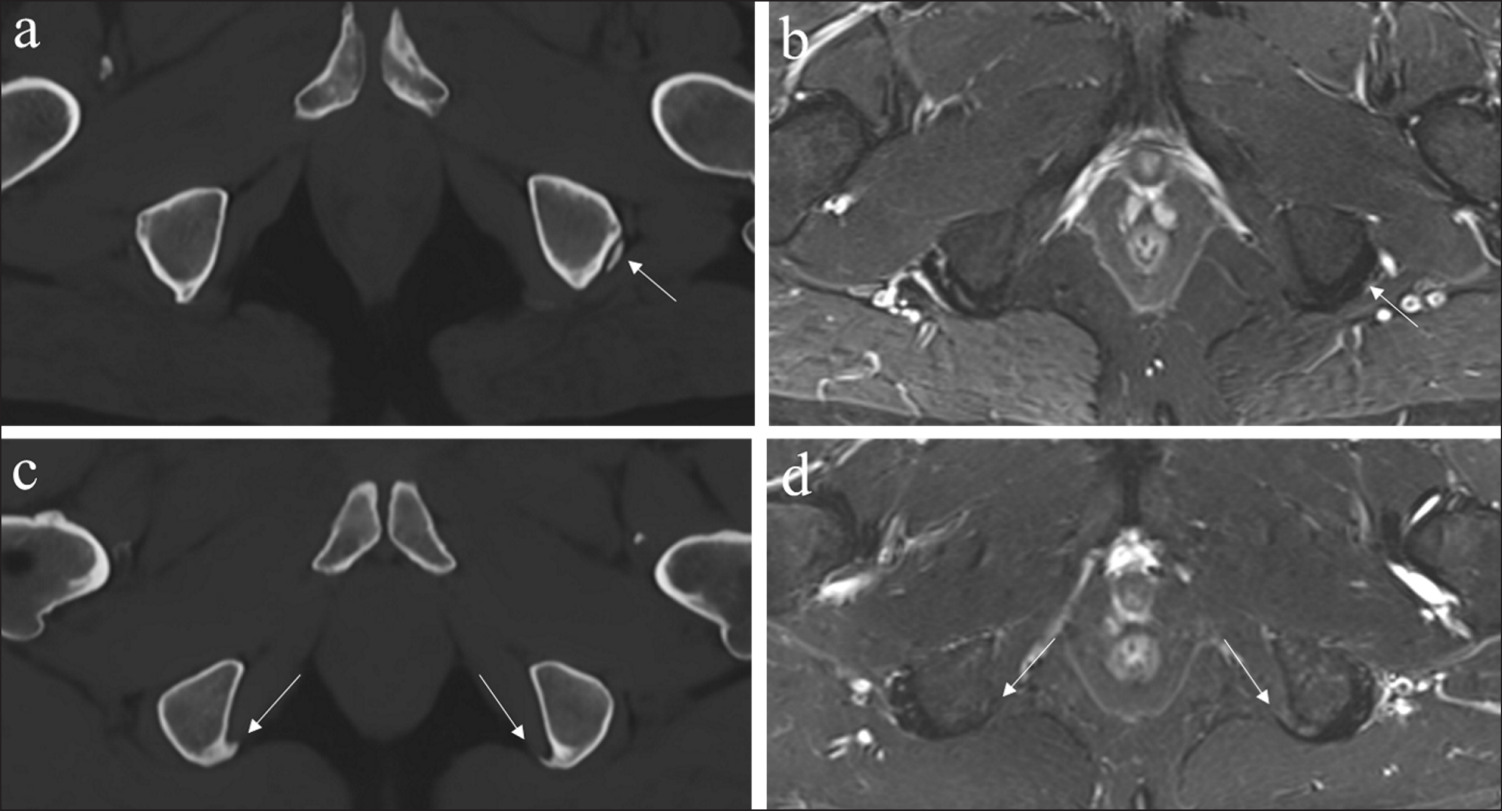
Incidental findings on sacroiliac joint MRI, depicted on synthetic CT images, but hard to appreciate on the T2 Short TI Inversion Recovery (STIR) images. (a, b) Old avulsion of the left ischial tuberosity in a 47-year-old male on synthetic CT (a), but hard to depict on the T2 STIR image (b). (c, d) Calcifications near the ischial tuberosities, suggestive of calcific enthesopathy, in a 35-yearold male with buttock pain, clearly visible on synthetic CT (c), but less evident on the T2 STIR image (d).
